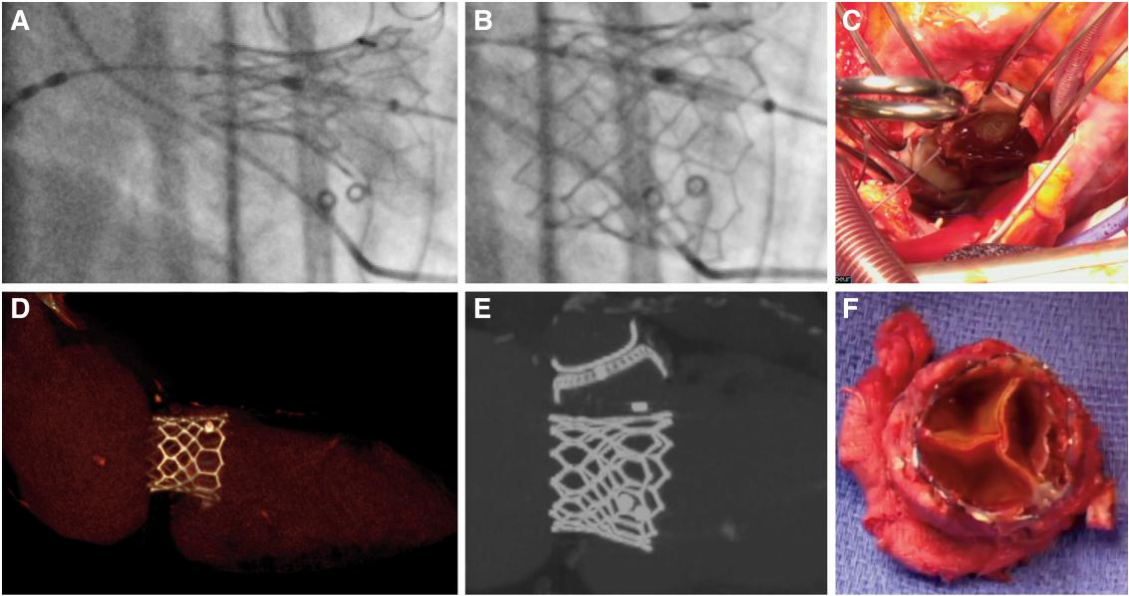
A comprehensive evaluation excluded infective endocarditis. Echocardiography confirmed prosthesis–patient mismatch, while gated cardiac computed tomography ruled out leaflet thrombosis or hypoattenuated leaflet thickening. Instead, incomplete valve expansion, which is common in valve-in-valve procedures, was identified as the cause of the elevated gradients. This phenomenon arises when the surgical valve ring constrains the transcatheter heart valve (THV), resulting in suboptimal expansion (*Panels D* and *E*), reduced effective orifice area, and impaired haemodynamics. Although this issue is well recognized in aortic valve-in-valve cases, similar findings are less documented in mitral transcatheter mitral valve replacement (TMVR).
